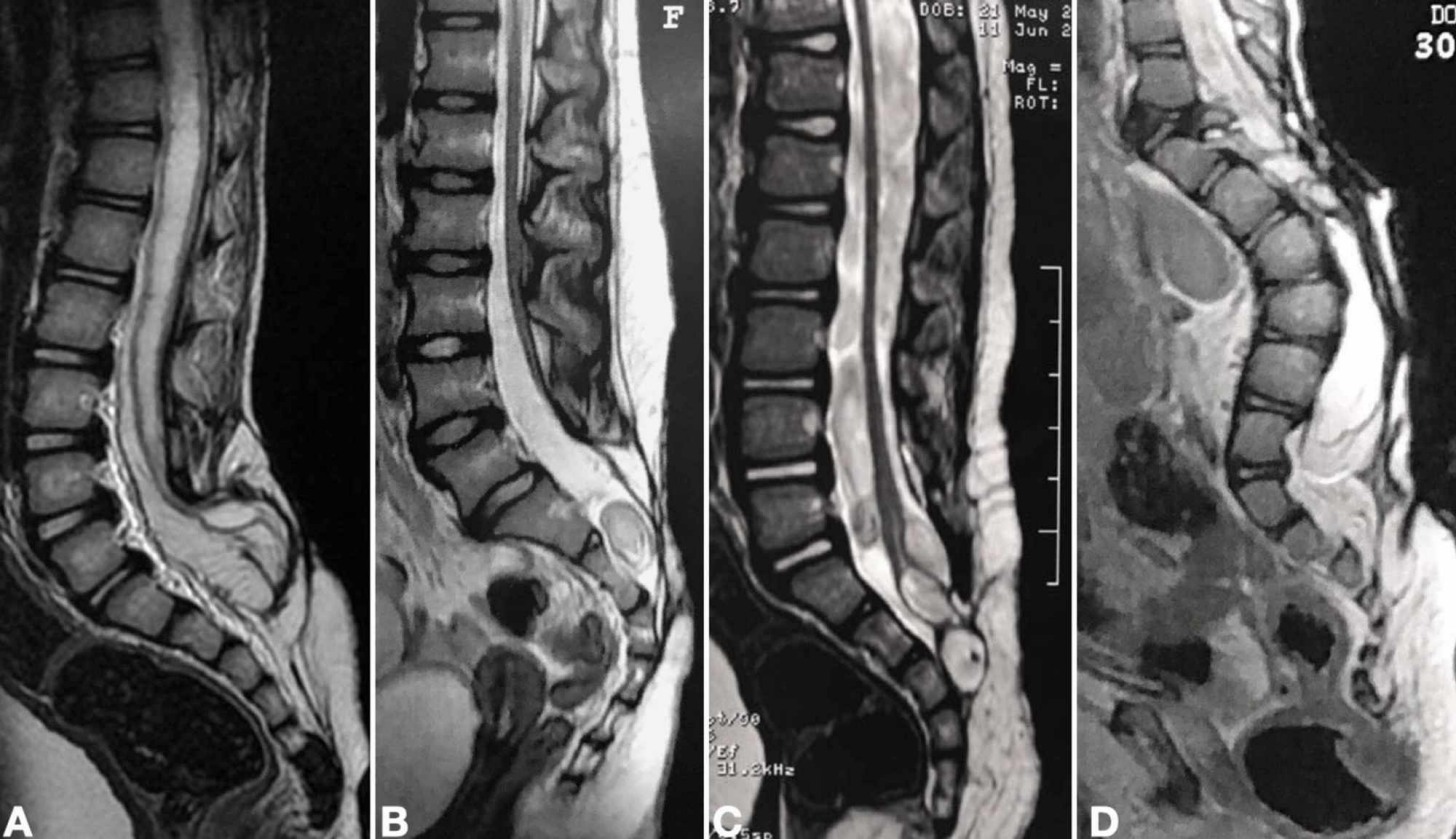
Features of several radiological tethering levels in children presenting with tethered cord syndrome after MMC repair Sagittal T2-weighted spinal cord MR image displaying severe syringomyelia with a large adherence of placode, classified as grade II (A). Patients in B and C presented as grade III. Low conus position was associated with a dermoid cyst in an MMC repaired postnatally (B) or exhibited two intramedullary epidermoid cysts in a child who underwent fetal repair of MMC (C). Lumbar kyphosis is associated with a low conus in grade IV (D) MMC: myelomeningocele
